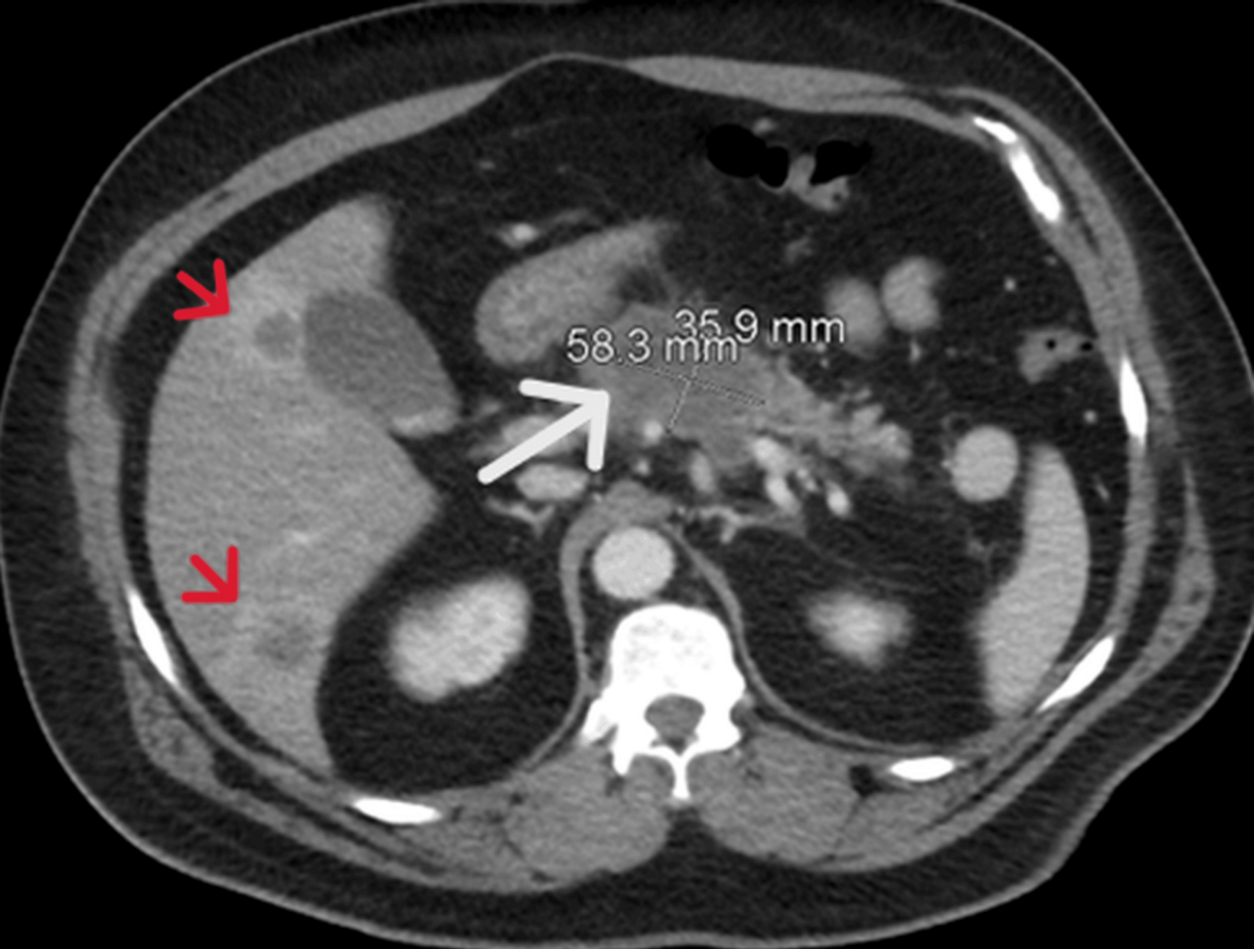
Computed tomography (CT) scan of the abdomen with contrast, revealing a hypodense pancreatic mass (white arrow) and multiple enhancing low-density liver lesions (red arrows)
